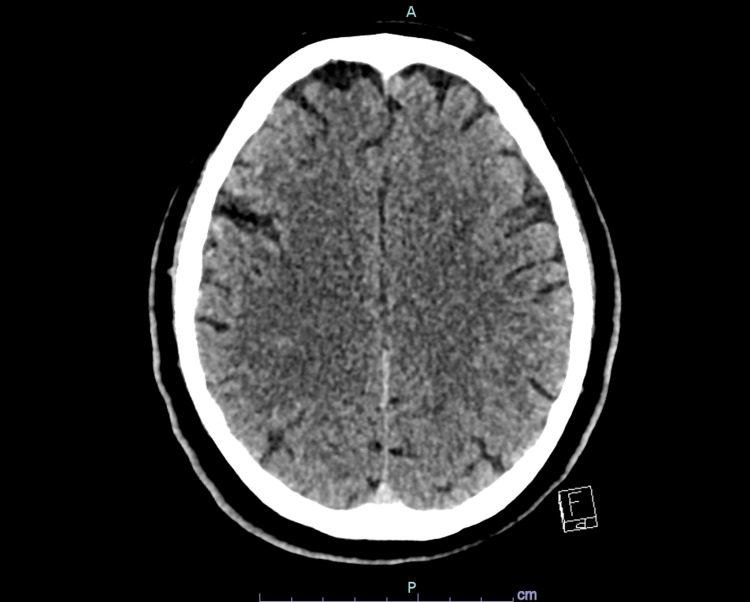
Non-contrast axial CT brain at a high convexity level demonstrating no acute intracranial haemorrhage or infarction
